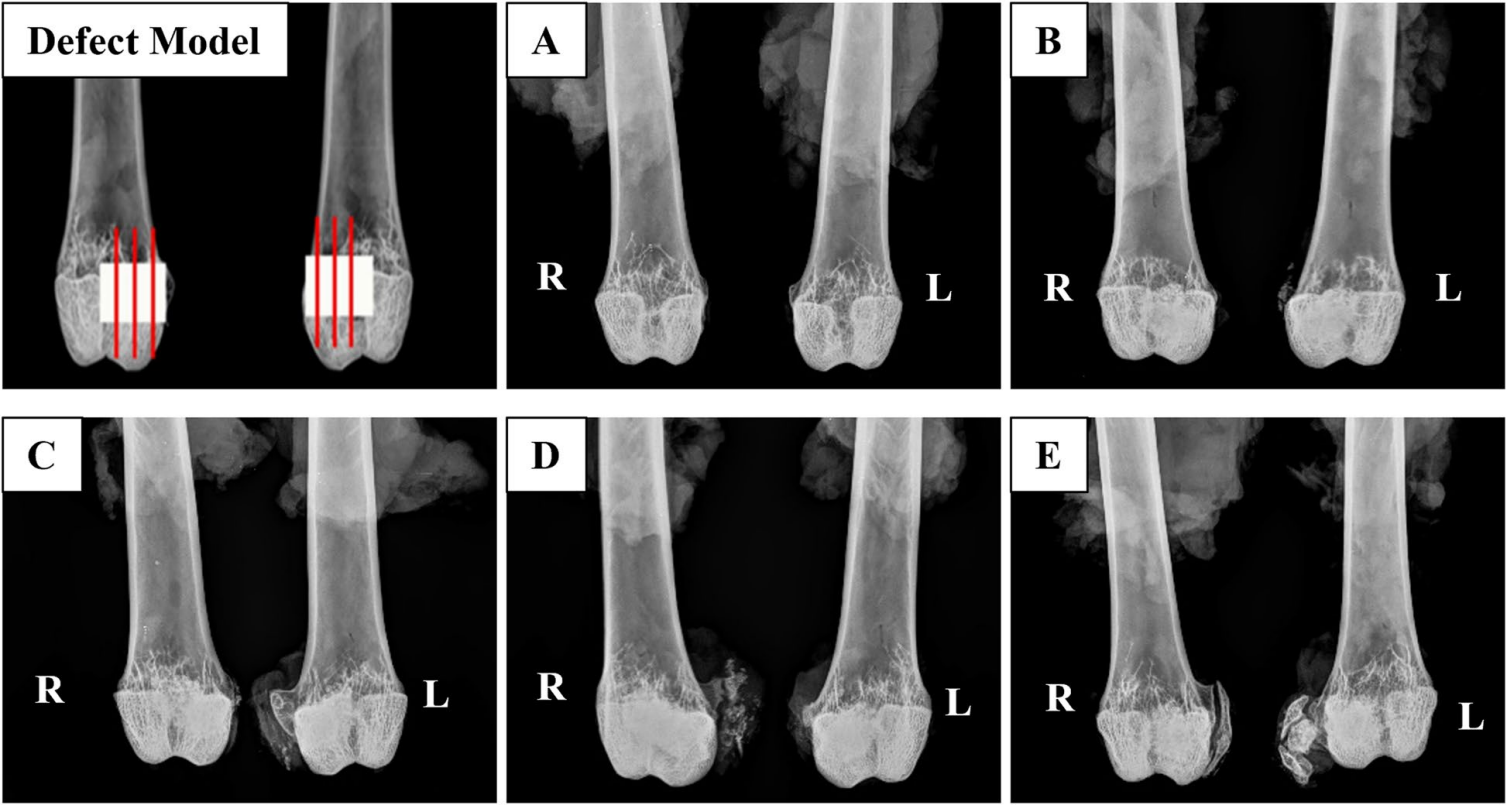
Representative Faxitron radiographs at 12 weeks. A Left: Empty defect, Right: Autograft, B Left and Right: MCaP carrier control, C Left and Right: MCaP carrier with rhBMP-2 (0.04 mg/cc), D Left and Right: MCaP carrier with rhBMP-2 (0.16 mg/cc), E Left and Right: MCaP carrier with rhBMP-2 (0.6 mg/cc)
